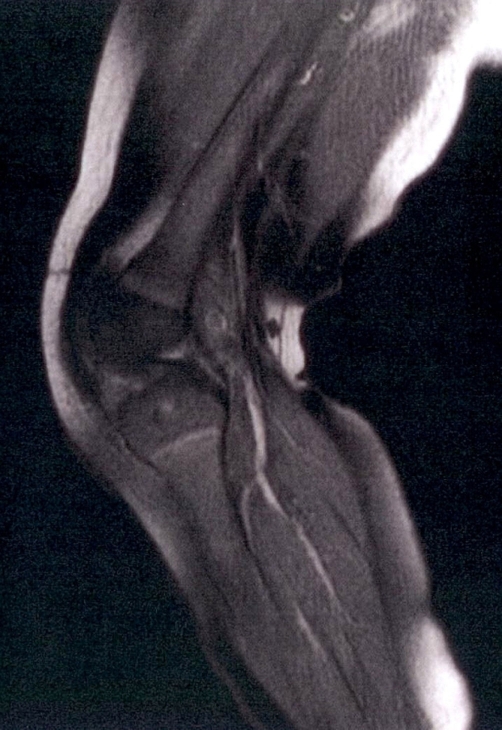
Follow up MRI (T1-WI, FS and IV in sagittal projection) with remarkable improvement of imaging findings.
